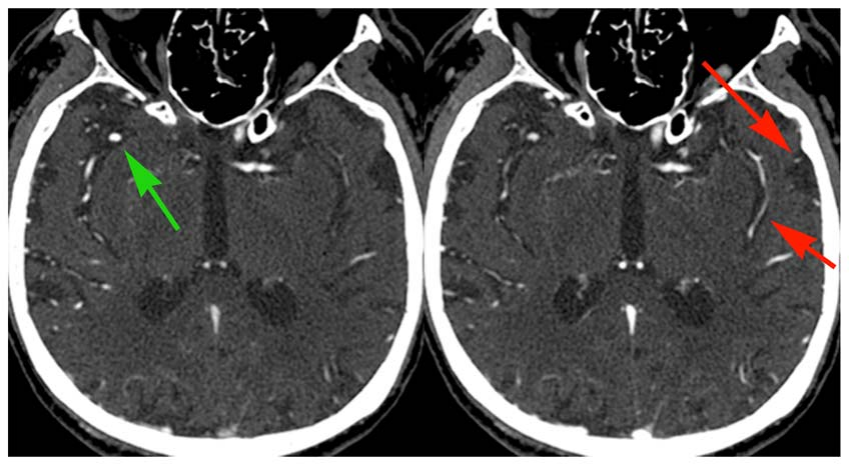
Example of delayed collateral vessels filling beyond a proximal occlusion. 83 year old male with atrial fibrillation and acute stroke. Early phase images show occlusion of the proximal M1 segment of the left MCA and robust opacification of the anterior division of the right MCA (green arrow). Delayed phase images show opacification of several pial vessels over the left temporal convexity and in the left Sylvian fissure (red arrows), delayed because of pial-pial collateral flow beyond the occlusion. Note that normal vessels demonstrate reduced opacification compared with the initial phase images (green arrow); this is due to decreased contrast concentration as a result of recirculation of the initial bolus. This CTA was obtained after MRI demonstrated the extent of MCA territory infarction; no management decisions were changed as a result of the delayed views.
